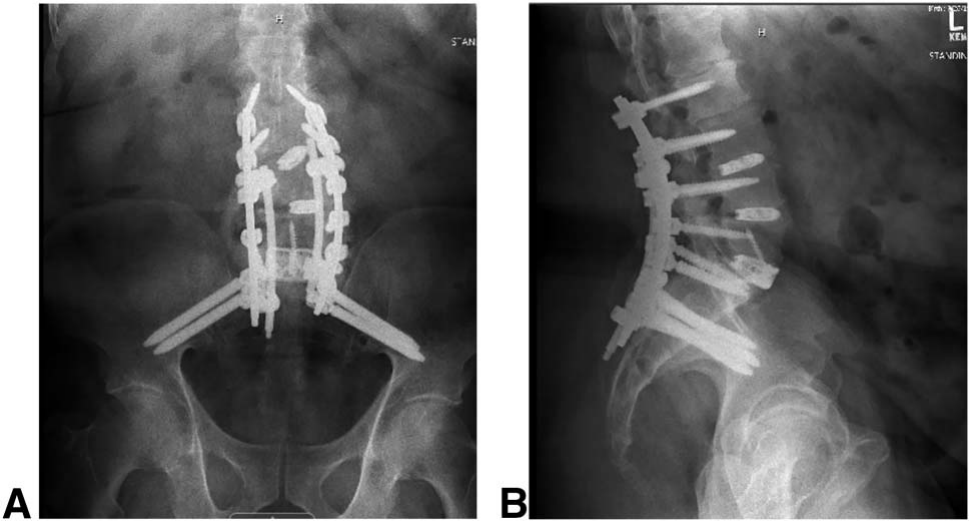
A, AP and (B) lateral radiographs of the lumbar spine showing replacement of infected L5-S1 transforaminal lumbar interbody fusion with an anterior lumbar interbody fusion cage.
